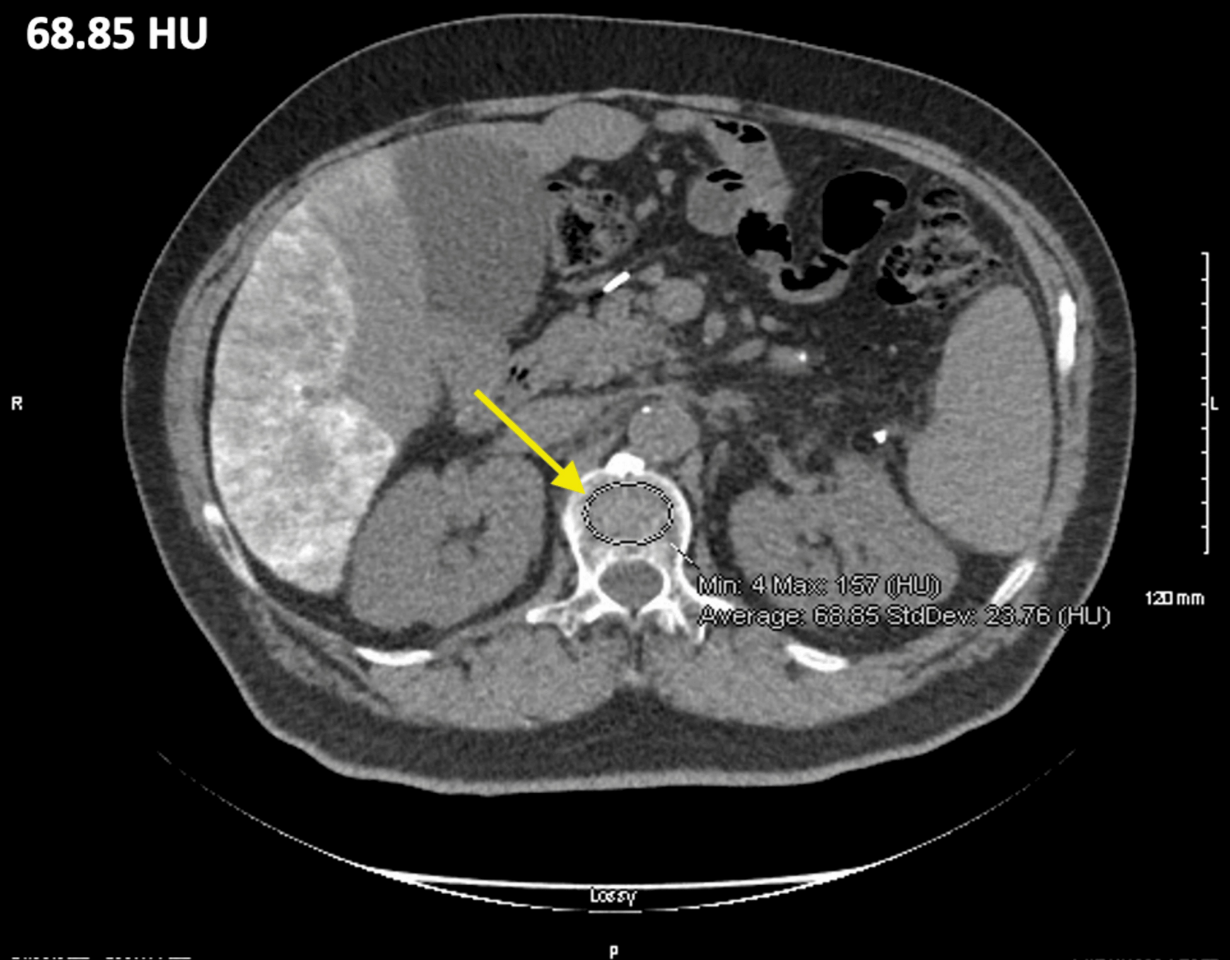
L1 Vertebra of an HCC patient The unenhanced axial abdominal CT scan of the L1 vertebra (arrow) in a 58-year-old male patient with HCC shows a measurement of 68.85 HU. HCC: Hepatocellular carcinoma, HU: Hounsfield units
